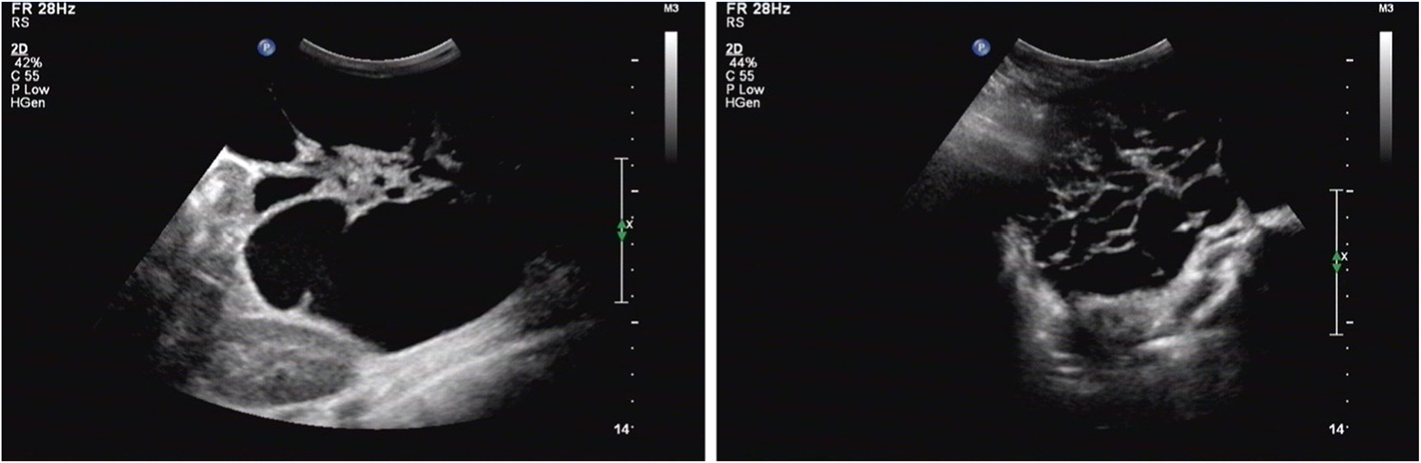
Ultrasonography displayed several echogenic areas in strip or tubal form communicating with each other in the lower abdomen and internal spotted medium echoes.
